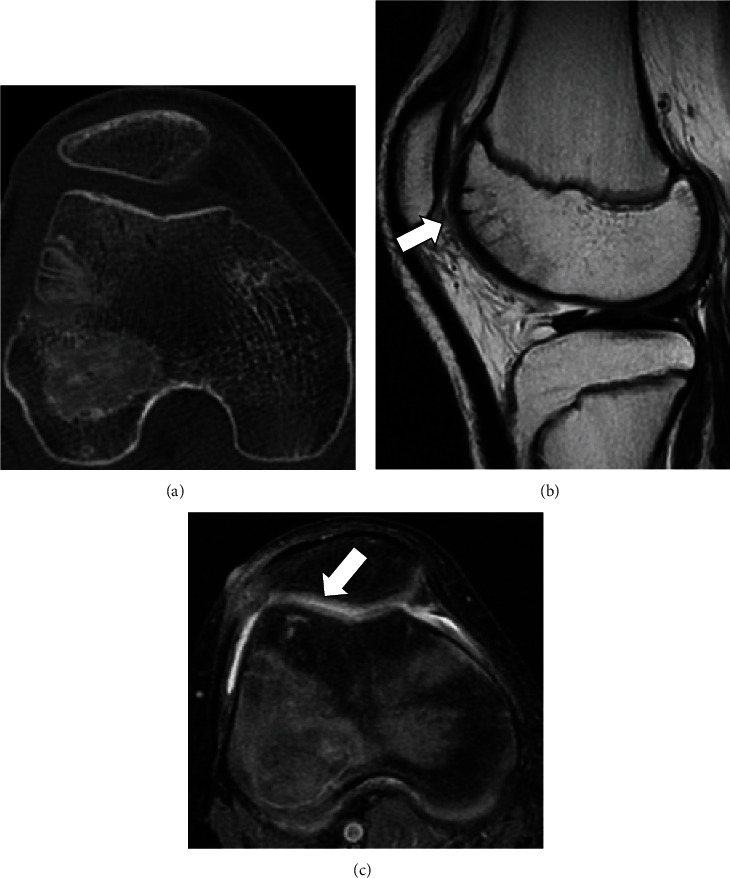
Postoperative axial (a) computerized tomography and sagittal (b) T2-weighted and axial (c) T2-weighted, fat-suppressed magnetic resonance image showing a smooth, healing chondral surface with osteochondral peg in place 2 months after osteochondral peg fixation.
